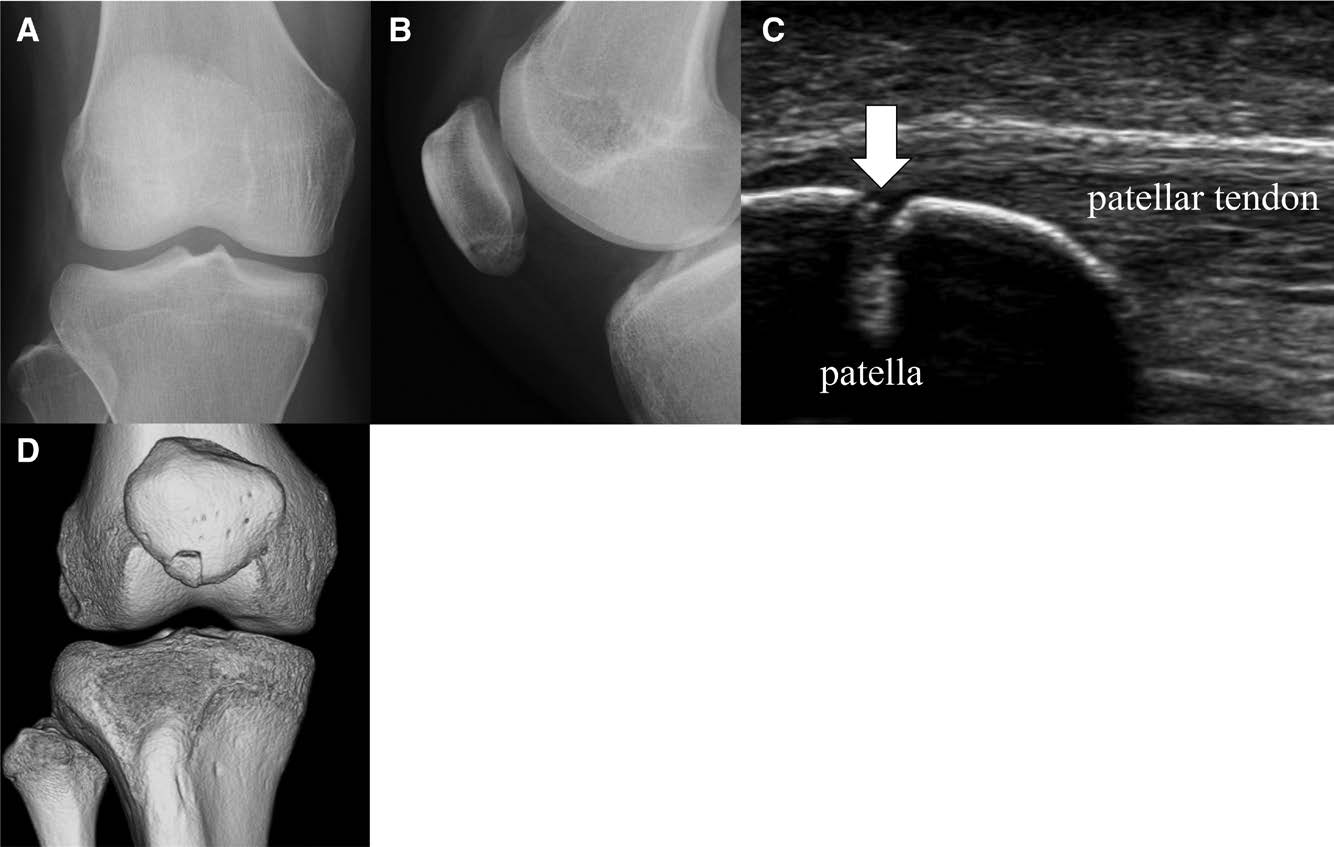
Imaging findings at the initial examination. (A, B) Radiographs. (C) Ultrasound. White arrow represents fracture. (D) Three-dimensional computed tomography.
